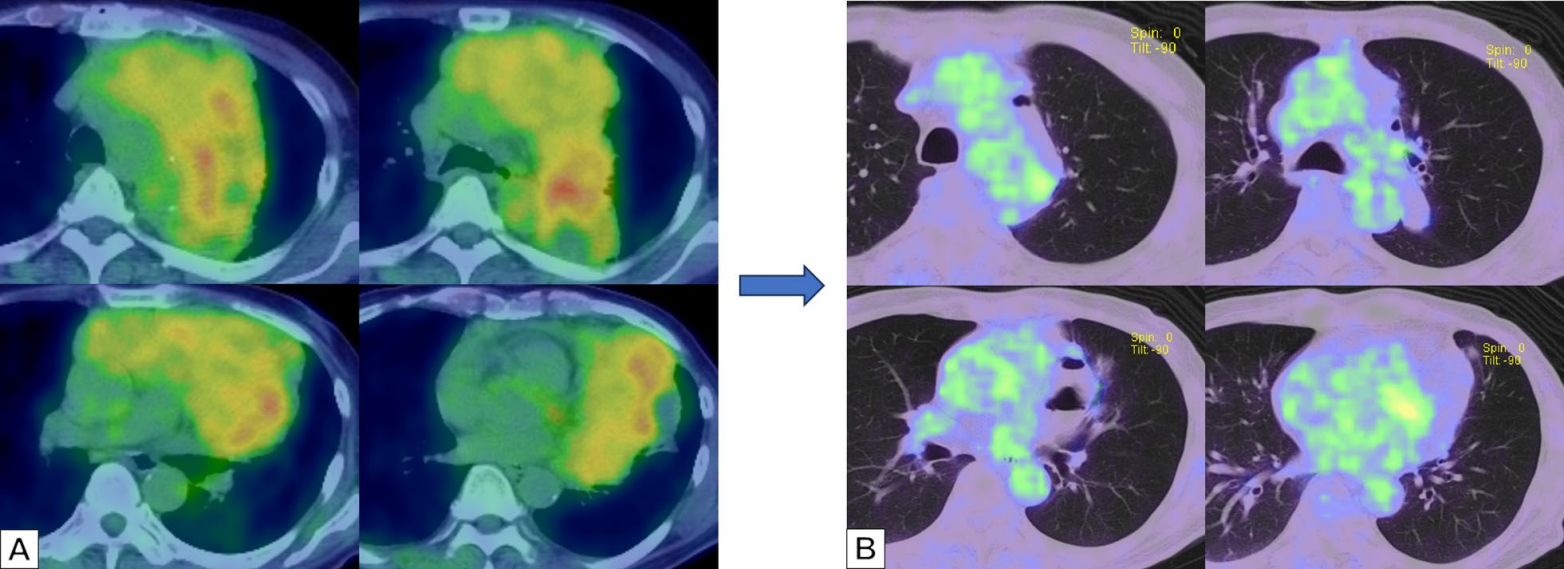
A Positron emission tomography/computed tomography image taken before treatment revealed increased ^18^F-fluorodeoxyglucose uptake (standard uptake max value 4.07) at the tumor site. B Positron emission tomography/computed tomography image taken after four cycles of preoperative induction chemotherapy with cisplatin, doxorubicin, and methylprednisolone (CAMP). Tumor ^18^F-fluorodeoxyglucose uptake decreased (standard uptake max value to 2.75)
